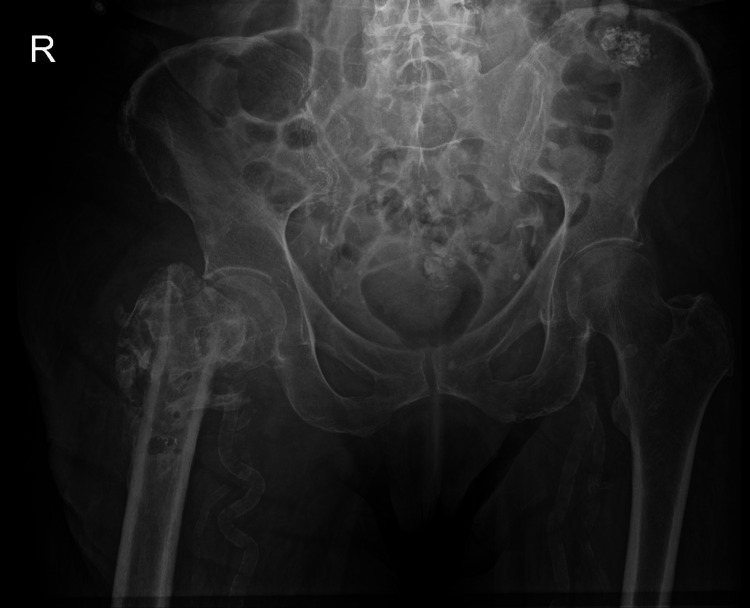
Pre-operative anteroposterior pelvis X-ray demonstrating right proximal femoral remodelling deformity An anteroposterior pelvic radiograph taken four months prior to surgery showing marked remodelling deformity of the right proximal femur with superior displacement of the distal fragment, consistent with chronic post-infective and post-fracture changes. No acute fracture or dislocation is evident. Mild degenerative changes are present within both hip and sacroiliac joints, with vascular calcification visible. The left hip joint remains preserved. These findings, in conjunction with persistent pain and mechanical instability, led to the decision to proceed with right proximal femoral replacement.
